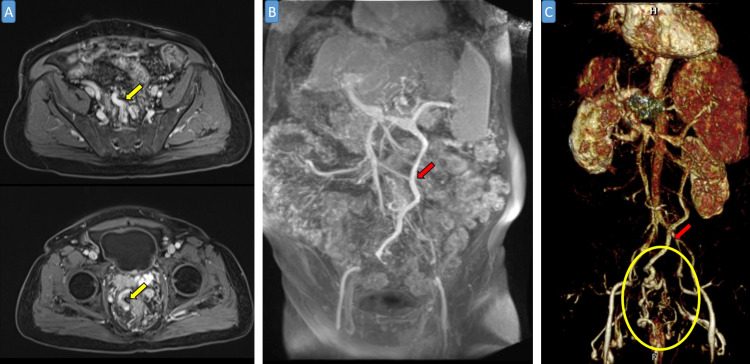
Abdominal MR venography: axial T1-weighted imaging post-contrast (A), coronal abdominal MIP reformat (B), and coronal 3D reformat (C). Imaging shows significant dilatation of perirectal varices (yellow arrows) as well as dilated tortuous inferior mesenteric vein (red arrows in B and C). The tortuous dilated inferior mesenteric vein in continuous with the rectal varices (yellow circle in C).
